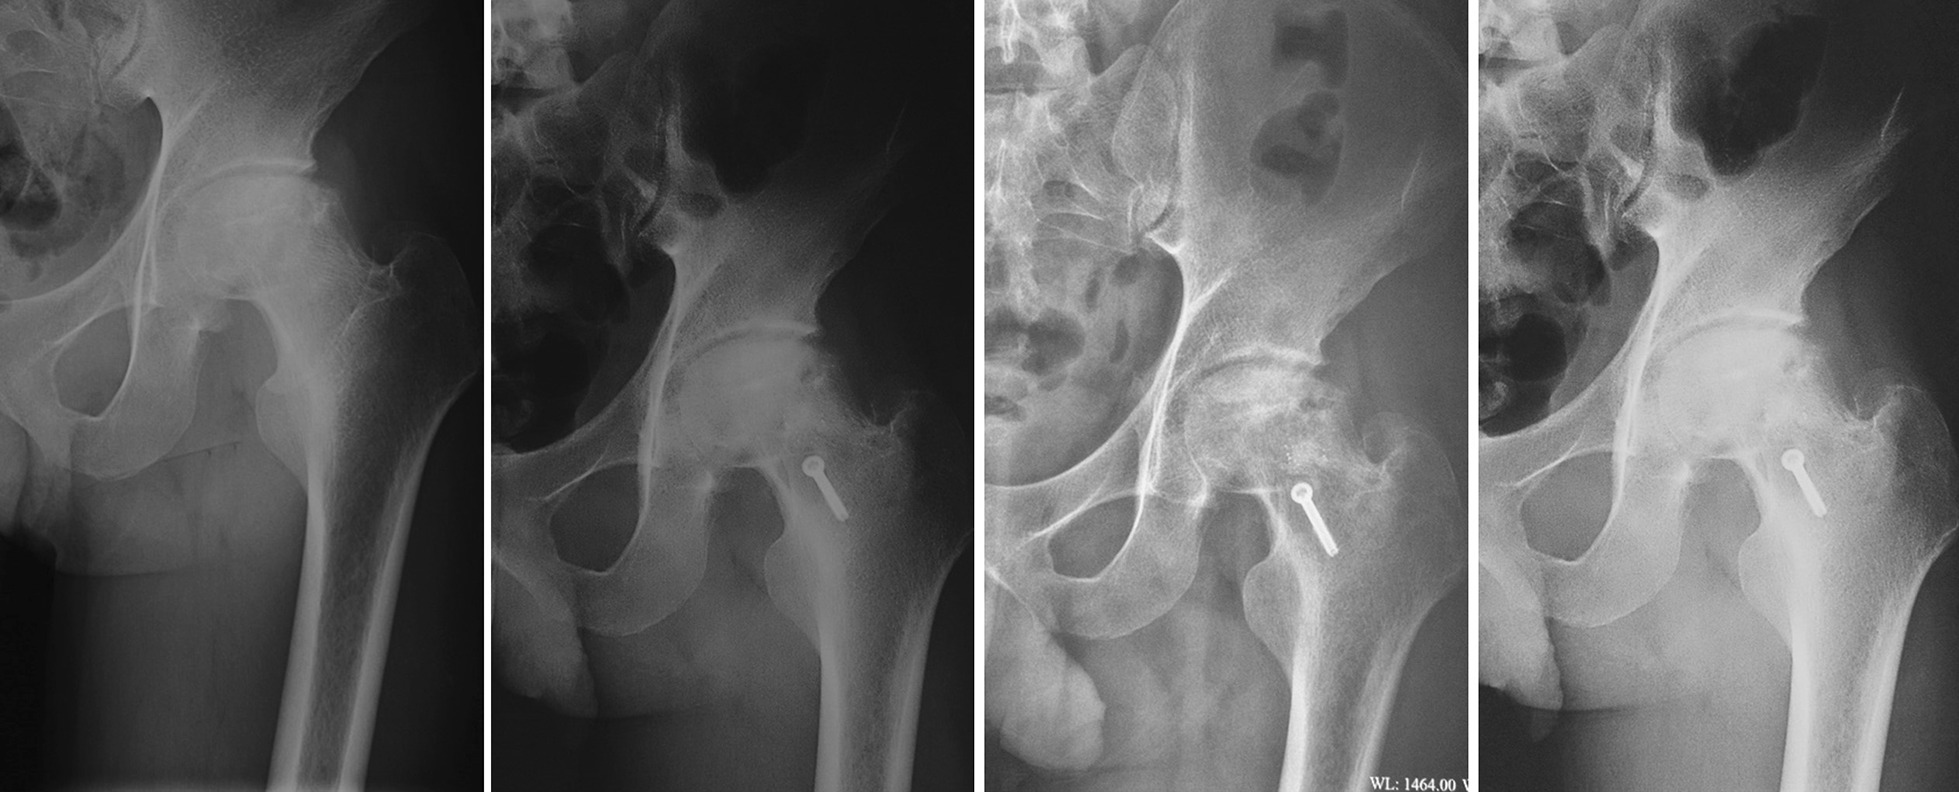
X-ray comparison of patients before and after the operation
